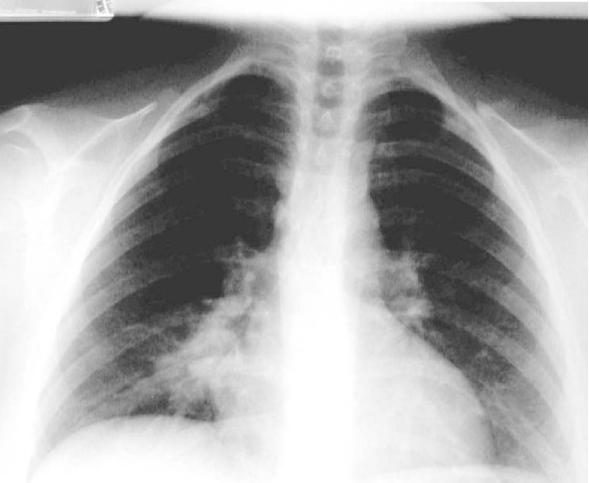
X-ray of the chest showing an infiltrate in the lower lobe of the right lung. Reproduced with permission from Al-Jahdali H [15]
